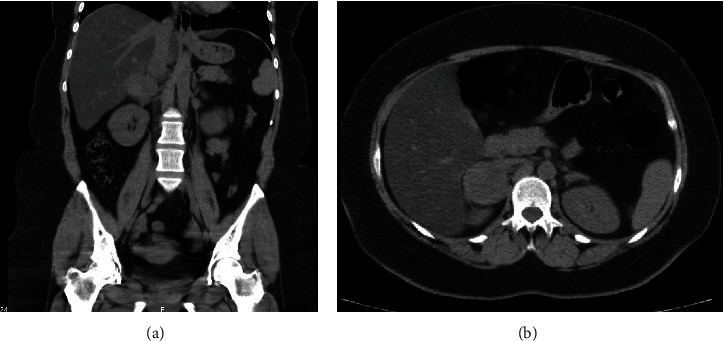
Case 2 abdominopelvic CT scan (at admission). The CT scan shows a 72∗66 mm bilobate mass of the right adrenal gland in coronal (a) and transverse (b) views.
